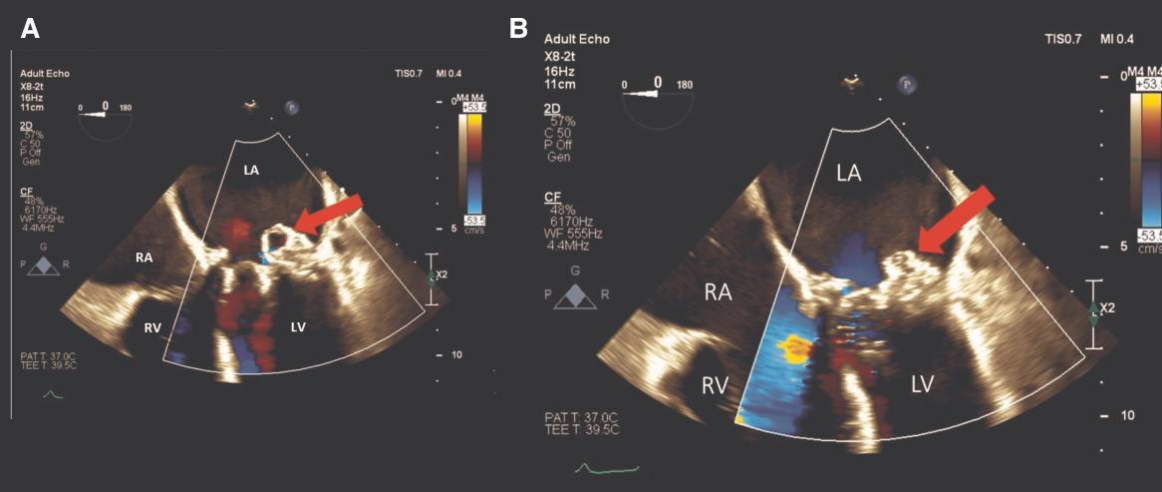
Two-dimensional transoesophageal echocardiography (midoesophageal four-chamber view) with colour flow Doppler demonstrates the bioprosthetic mitral valve abscess expansion in systole (*A*, arrow) and collapse in diastole (*B*, arrow). LA, left atrium; LV, left ventricle; RA, right atrium; RV, right ventricle.
